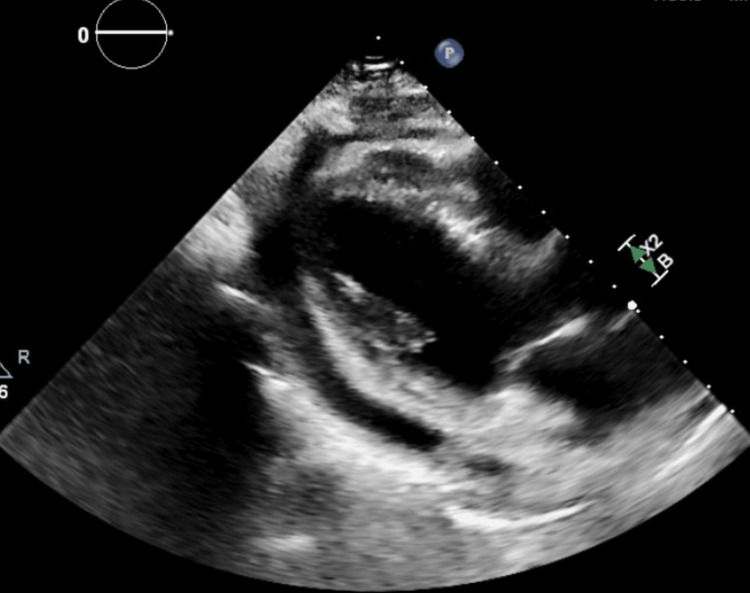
Echocardiogram Parasternal Long-Axis View The ECHO shows a moderate pericardial effusion measuring 12 mm without findings of tamponade physiology. Normal global systolic left ventricular function with ejection fraction estimated at 60-65% was noted. The right ventricular systolic pressure was 26 mmHg. ECHO: echocardiogram.
